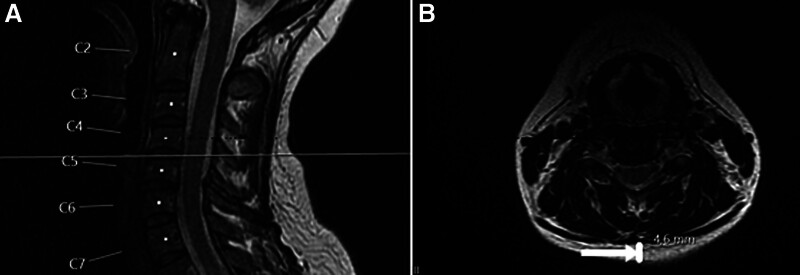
A 34-yr-old woman with neck pain. Cervical midsagittal T2-weighted image (A) and measurement of cervical subcutaneous fat tissue thickness in the axial T2 fast spin-echo image (B) passing through the C4-C5 intervertebral disc space (arrow).
